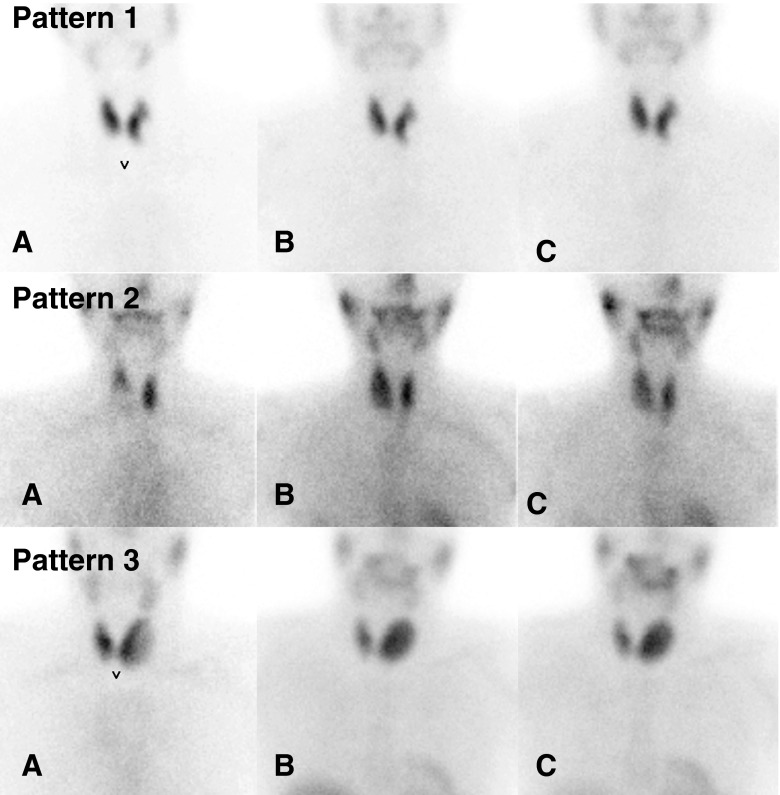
Visual assessment of ^99m^Tc-MIBI scintigraphy. a
